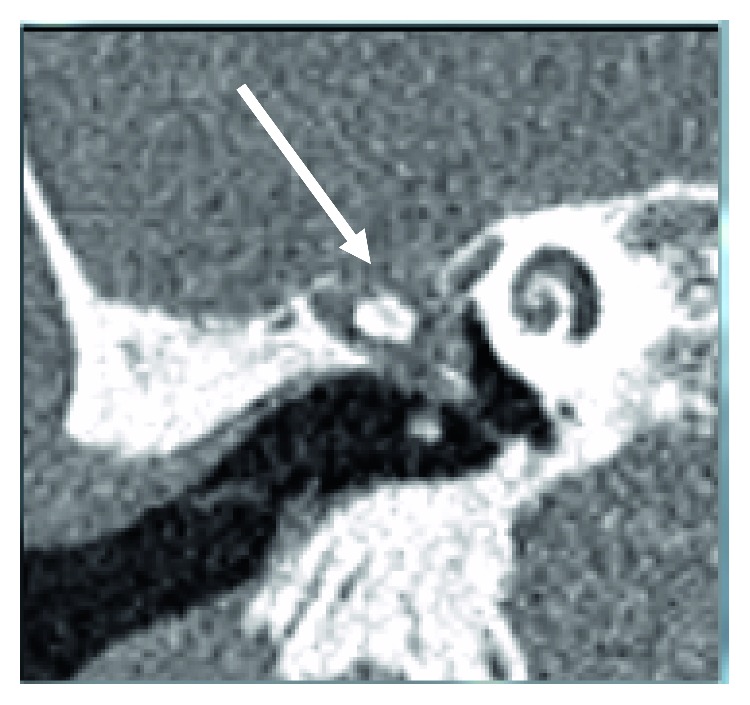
CT scan with the defect shown: high-resolution CT petrous bones (coronal view).
